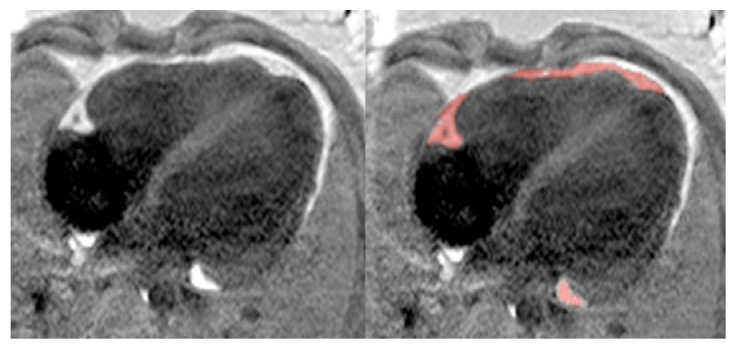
Epicardial adipose tissue identification by MRI. (Left) MRI phase-sensitive inversion recovery cardiac slice of an obese subject. (Right) Results from the semi-automatic growing region of interest method used to identify epicardial adipose tissue on this slice (red).
